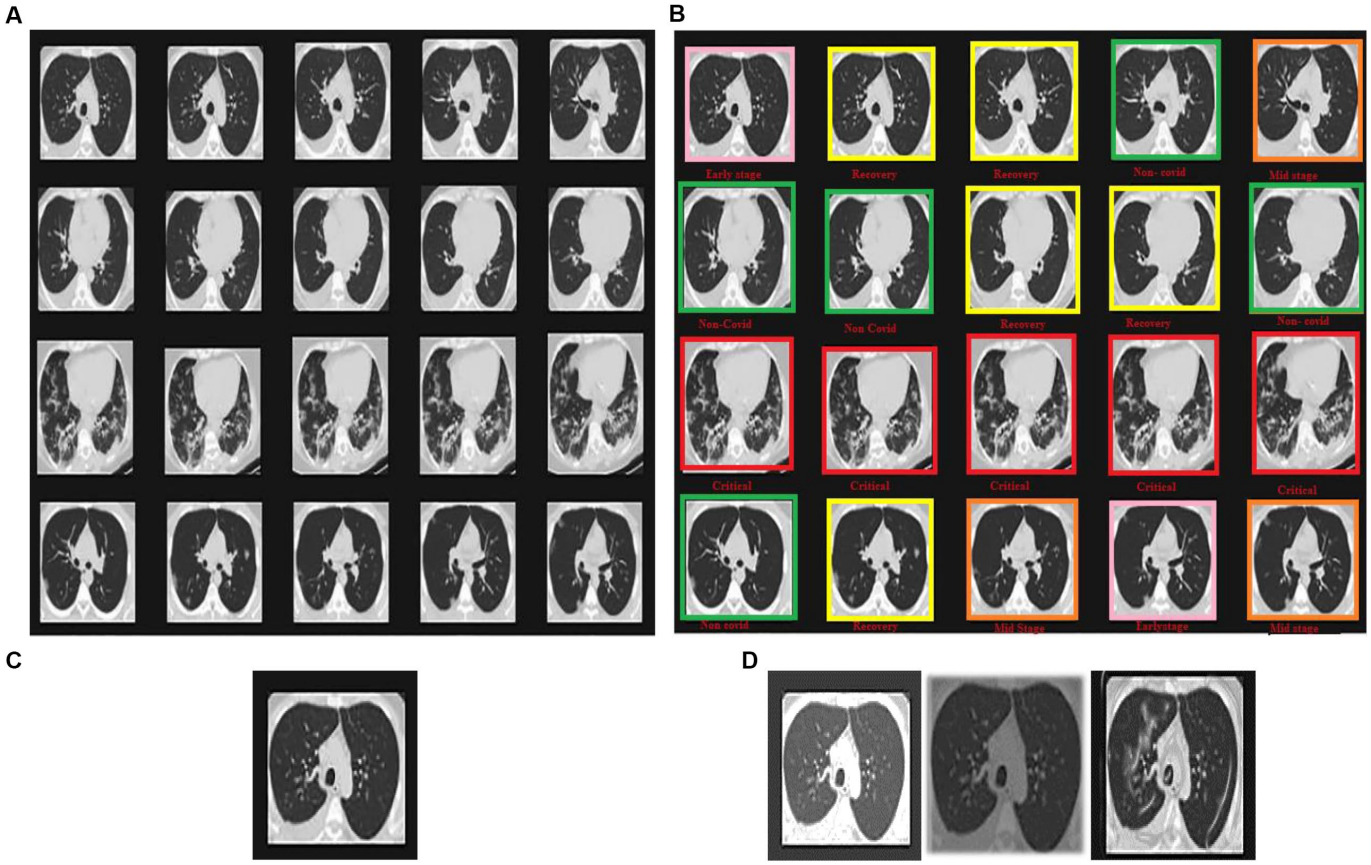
Sample images displaying lung CT images classification in terms of (A) 2D axial views, (B) COVID-19 severity classes, (C) original image, and (D) synthetic images of loaded, rescaled, and grayscale.
